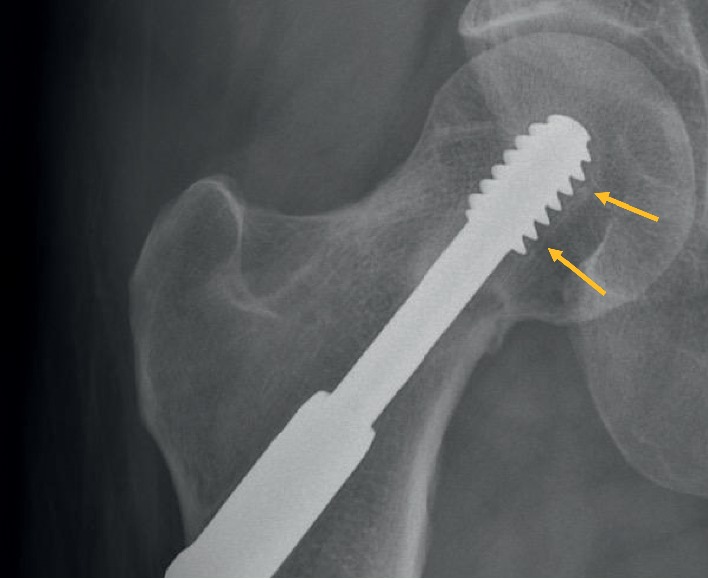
Right hip X-ray showing mobility around the DHS (arrows) and a fracture line on the compression side.
